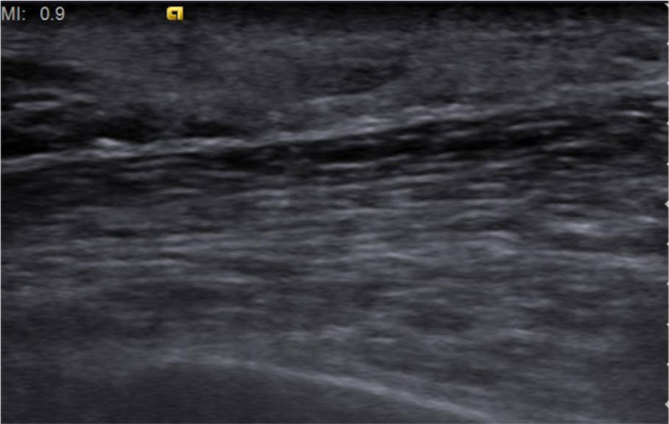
Follow-up ultrasound shows significant reduction in the size of the mass.
